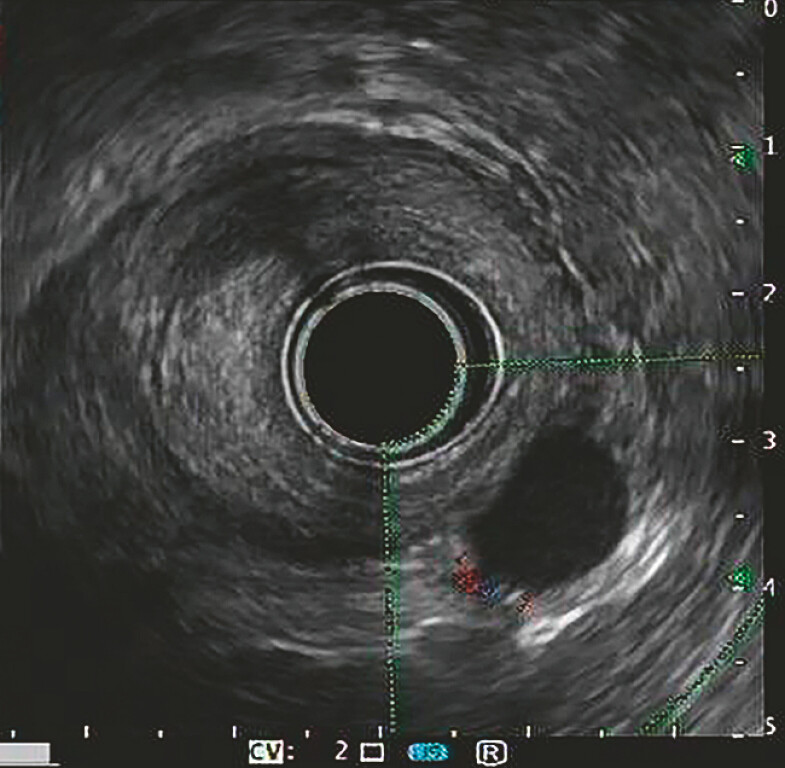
Endoscopic ultrasound imaging showed that the lesion originated from the muscularis propria and was located deep within it.
